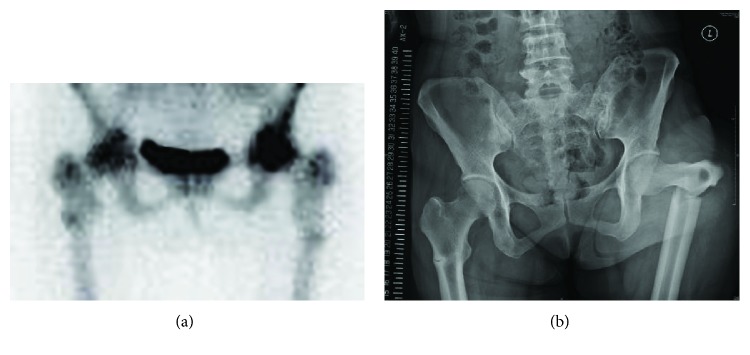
Preoperative images of bilateral atypical femoral fractures (AFFs). (a) Bone scintigraphy showing increased uptake in both proximal femurs at follow-up before a left displaced AFF. (b) Radiography showing a left displaced AFF and a right incomplete AFF.
